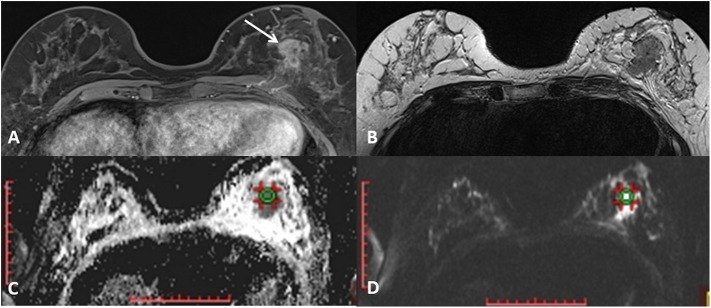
A mass lesion is identified on the first post-contrast T1-weighted image (A) and the absence of cystic parts is confirmed on T2-weighted axial image (B). ROI of 1cm^3^ is defined on correspondent DWI image (C) and the ADC values are noted (D).
